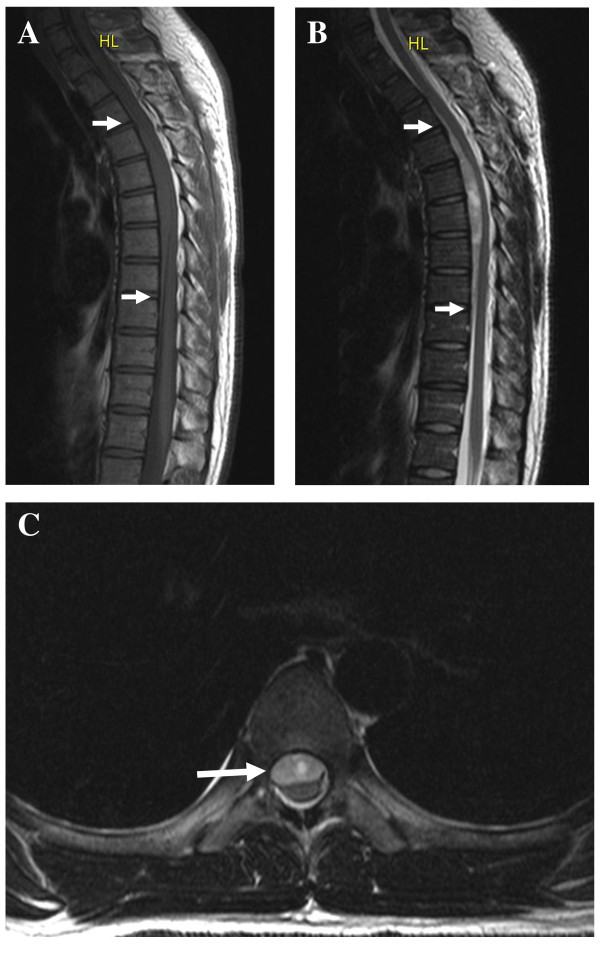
Magnetic resonance imaging of the thoracic spine. A, Sagittal T1-weighted imaging revealed an isointense anterior epidural mass extending from T3-T4 to T8-T9 (white arrows at the extremities of the mass), which compressed the spinal cord posteriorly, especially at the T5-T6 level. B, Sagittal T2-weighted imaging was helpful for delineation of the mass (white arrows at the extremities of the mass), which showed hyperintensity in this scan. The length was the same as that visualized with T1-weighted imaging, but the severity of the spinal cord compression was better visualized. C, Axial T2-weighted imaging revealed the epidural mass (white arrow) located in the anterior aspect of the spinal canal, compressing the spinal cord and obliterating the liquoric space.
